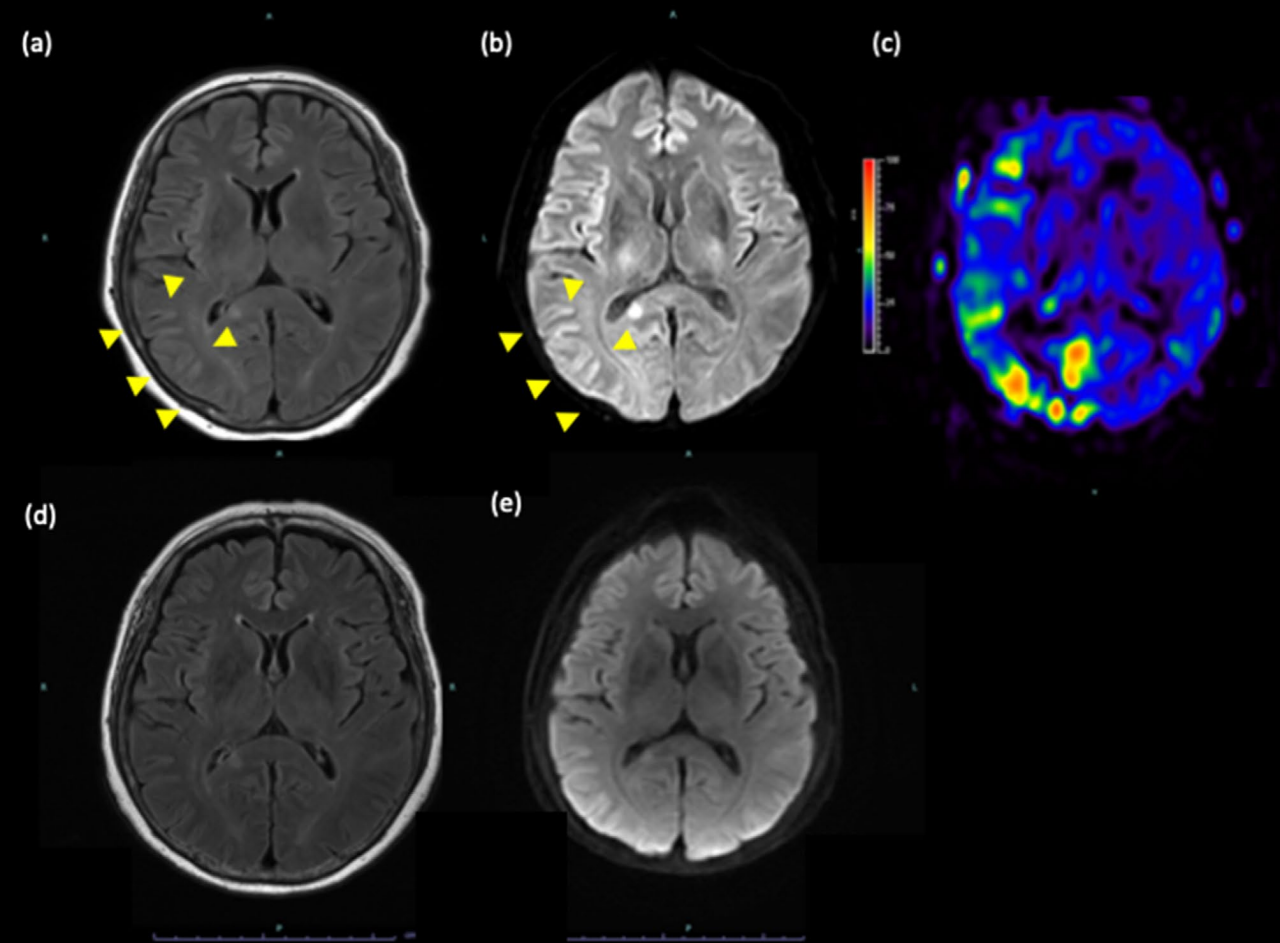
Magnetic resonance images. (a) T2‐Fluid Attenuated Inversion Recovery (FALIR); (b) diffusion‐weighted imaging (DWI); (c) arterial spin labeling (ASL). Initial magnetic resonance imaging revealed hyperintense vasogenic edema in the right occipital and parietal lobes (yellow arrows). Follow‐up magnetic resonance imaging revealed improvement of vasogenic edema on Day 17. (d) FALIR; (e) DWI.
